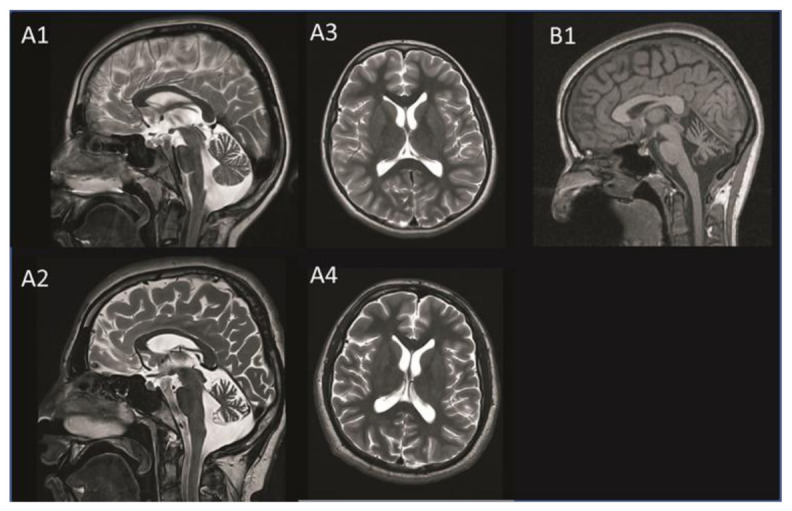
MRI images of patient A taken at age 11 (A1 + A3) and age 16 (A2 + A4) and those of patient B taken at age 20 (B1). Progressive cerebellar atrophy is visible on the T2-weighted saggital MRI image of patient A (A1 + A2). The transversal MRI image shows progressive mild cerebral atrophy without white matter lesions in patient A (A3 + A4). Cerebellar atrophy is visible on the T1-weighted saggital MRI image in patient B (B1). MRI, magnetic resonance imaging.
